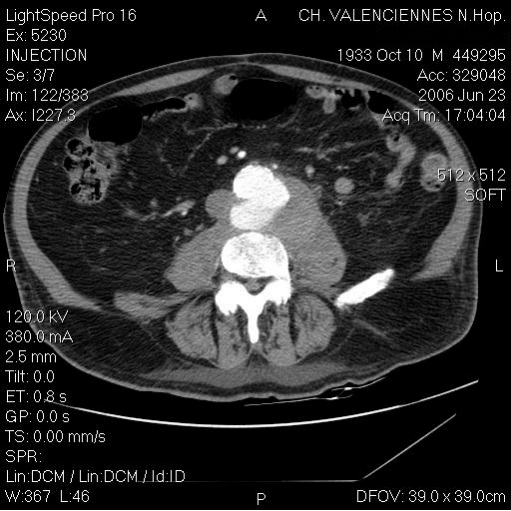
An axial CT image (the first to be performed for this patient, at the General Hospital, Valenciennes-France) with contrast of the lumbar area showing abdominal aortic aneurysm.
